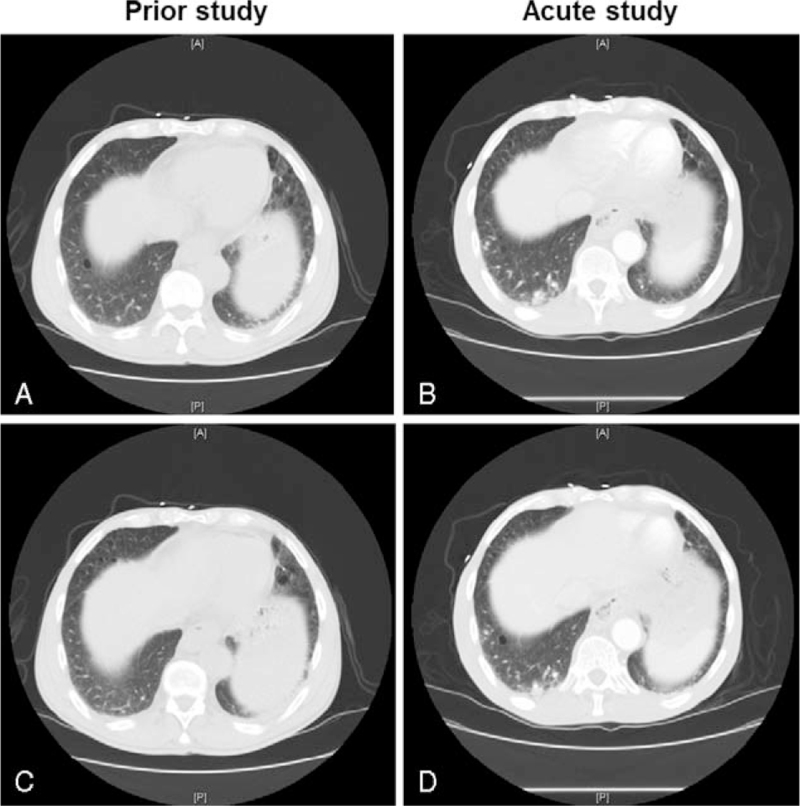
Chest imaging of *B bronchiseptica* pneumonia. Computed tomographic slices show similar levels (A & B, C & D) from a study obtained one year prior to the acute illness (A, C) compared with the study during the acute illness with *B bronchiseptica* (B, D). Images show nodular opacities in the right lower lobe.
